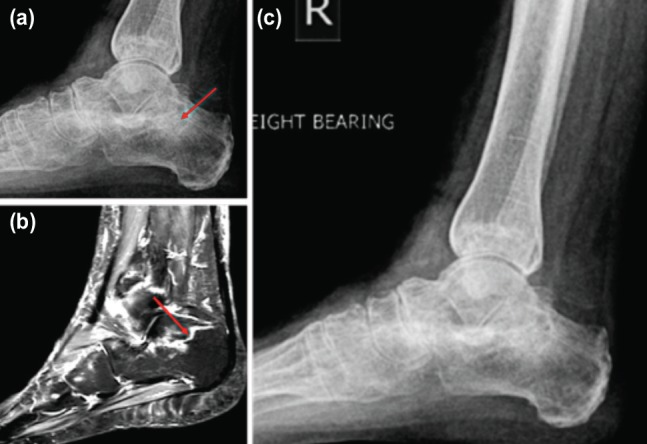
(a) Plain radiograph of the calcaneus with a suspicious fracture. (b) T2 weighted MRI images of the calcaneus showing insufficiency fracture. (c) Plain radiograph of the calcaneus showing the healed fracture.
